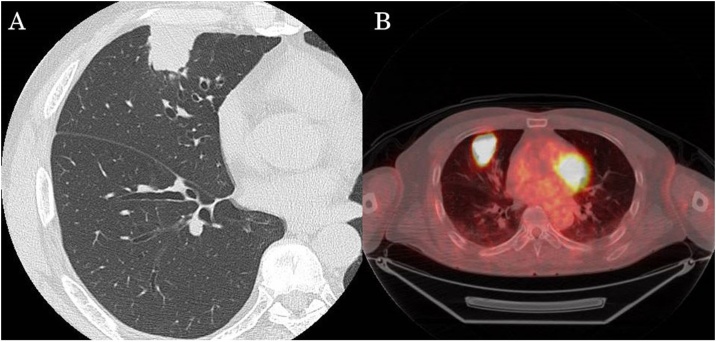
Radiographic findings of the tumor. *A*, Chest computed tomography revealed a 3.6-cm mass in the right peripheral S^5^ segment. *B*, On positron emission tomography, the mass showed ^18^F-fluorodeoxyglucose accumulation with a maximum standardized uptake value of 14.0.
